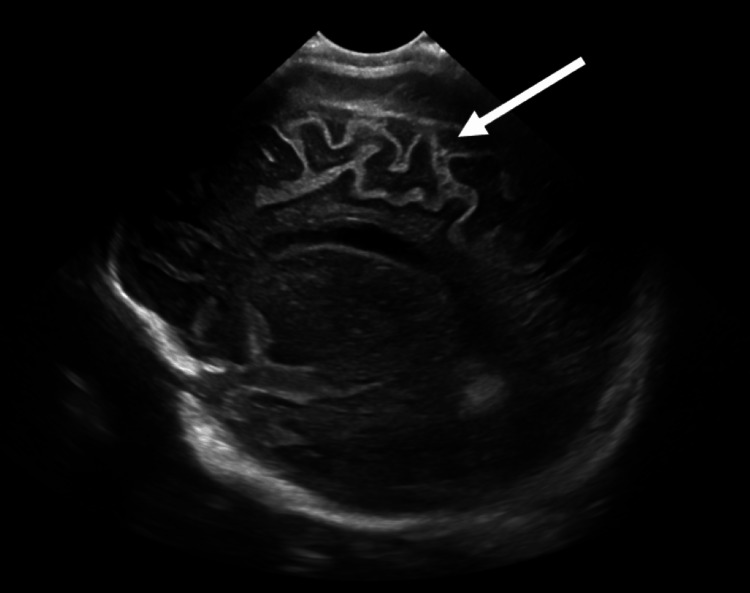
Head ultrasound, right sagittal view, demonstrates widened and echogenic cerebral sulci (arrow).
